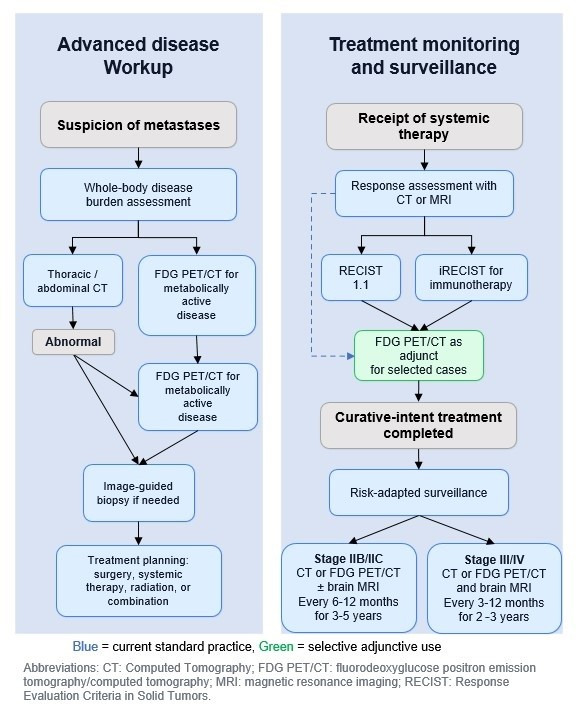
Clinical imaging pathway in cutaneous melanoma for treatment monitoring, surveillance, and advanced metastatic disease workup. Blue boxes represent current standard practice and green boxes represent selective adjunctive tools. Note: CT: computed tomography; FDG PET/CT: fluorodeoxyglucose positron emission tomography/computed tomography; MRI: magnetic resonance imaging; RECIST: Response Evaluation Criteria in Solid Tumors.
